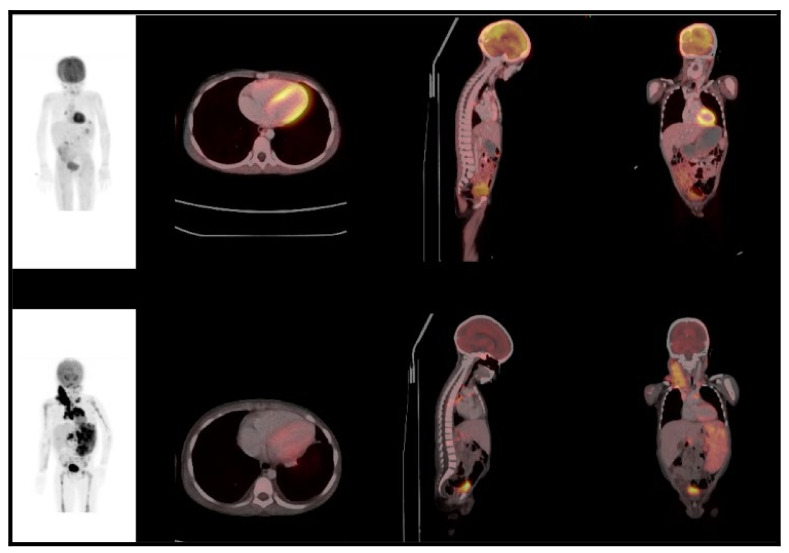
A 6-year-old male with Hodgkin lymphoma. Baseline images (bottom row: maximum intensity projection, MIP; axial, coronal, and sagittal fused PET/CT) demonstrated splenic and bone marrow involvement, as well as a pericardial effusion and small bilateral pleural effusions. tMTV-901.83 cm^3^; TLG-3655.06; SUVmax 10.78. After 6 cycles of ABVD (adriamycin, bleomycin, vinblastine, dacarbazine) chemotherapy, a partial metabolic response was noted on the end of treatment PET/CT (top tow*).* The patient later progressed with a PFS of 11.2 months.
